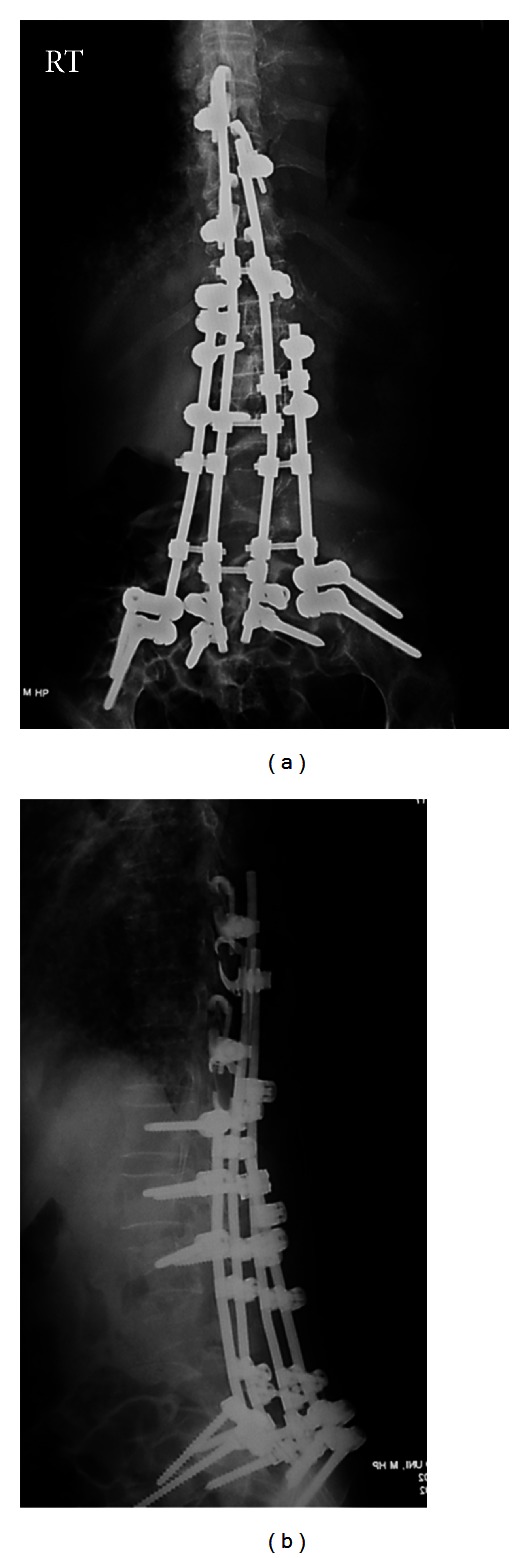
Plain radiographs after the second surgery (June 2010).
